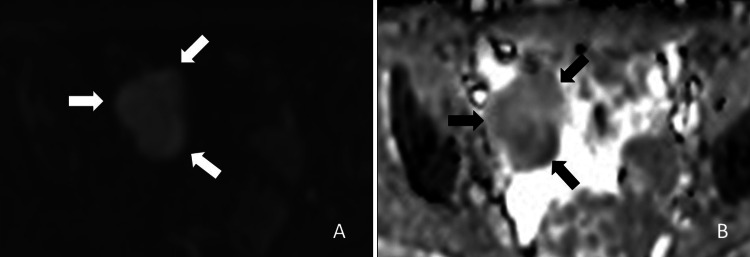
Magnetic resonance images, (A) diffusion-weighted image, and (B) apparent diffusion coefficient map. The adnexal mass shows mildly high signal intensity on the diffusion-weighted image (b = 1000 s/mm^2^) and heterogeneously high signal intensity on the apparent diffusion coefficient (ADC) map (ADC value = 1.12 × 10^-3^ mm^2^/s).
